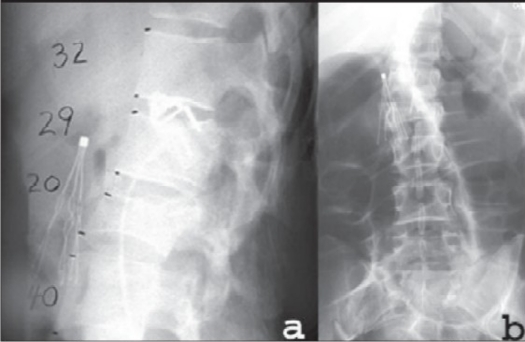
Lateral plain film radiograph (a) demonstrates a 17% loss of height at L1 and a 50% loss of height at L2. AP plain film (b) radiograph demonstrates a focal translation at the level of the L1-L2 subluxation causing a coronal plane deformity. An inferior vena caval filter is visualized
